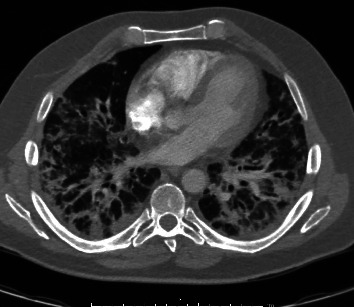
Chest CT showing bilateral airspace opacities and ground glass opacities.
